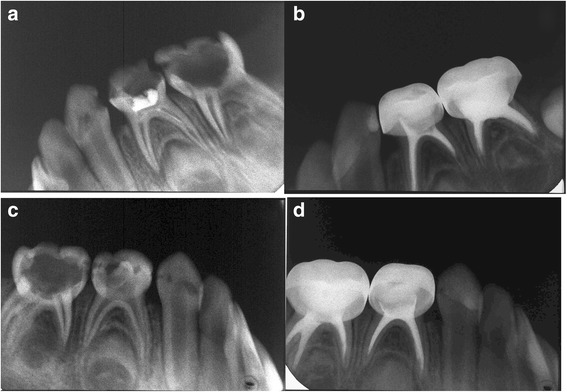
Eighteen months follow-up of MPRCF. a, c Pre–operative Radiograph of *right* and *left* mandibular first and second primary molar considered for root canal treatment with MPRCF. b, d Radiograph 18 months postoperatively showing the MPRCF fillings were stable and intact
